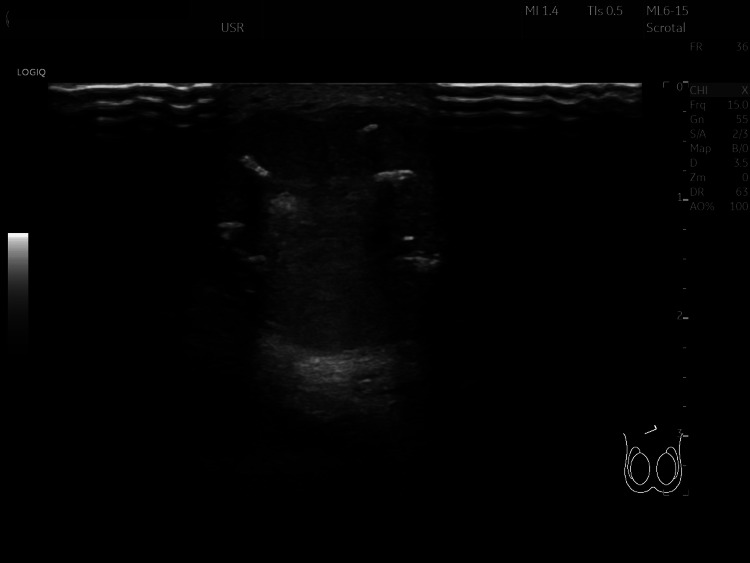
Ultrasound of soft tissue scrotal lesion
